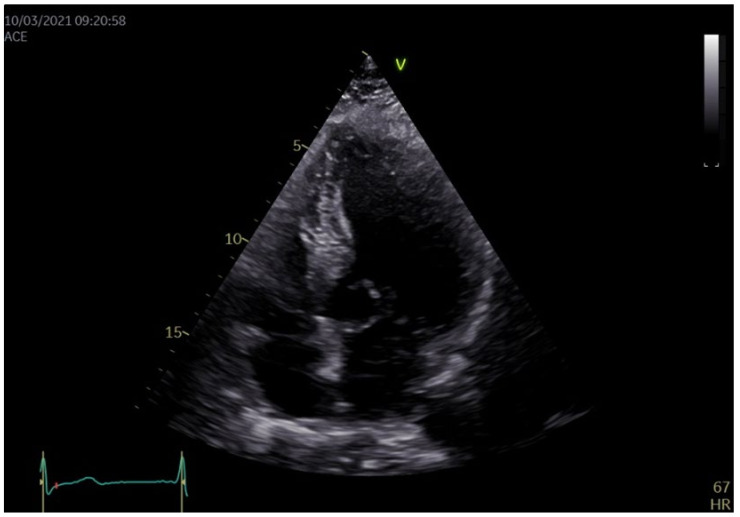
Transthoracic bidimensional echocardiography, 4 chamber view: a mitral valve with fibrosed leaflets, an elongated AML and SAM of both AML and PML, creating an anteriorly deviated “funnel”.
